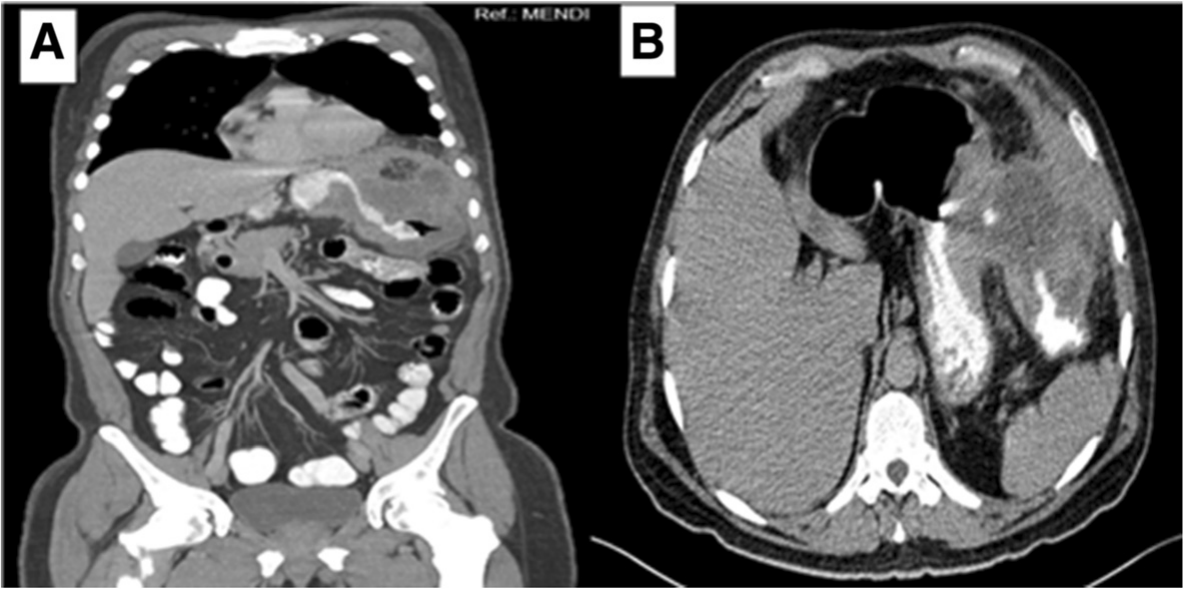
a Coronal computed tomography image showing a gastrocolic fistula secondary to a tumor of the splenic flexure of the colon infiltrating the greater curvature of the stomach and diaphragm. b Computed tomography image of the abdomen showing passage of contrast material through a fistulous connection between the stomach and the splenic flexure of the colon
